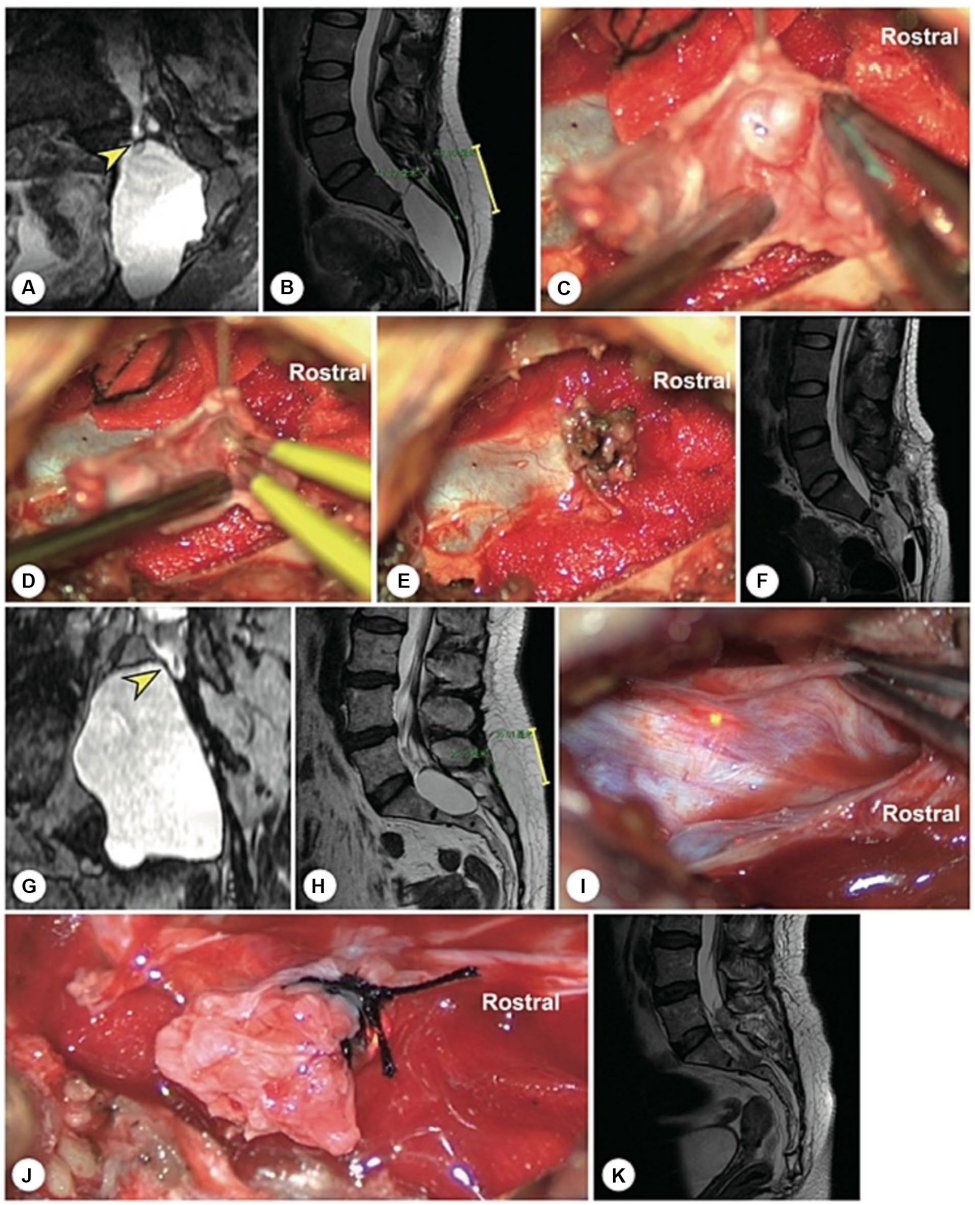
A representative case of an arachnoid hernia-type cyst and a representative case of a spinal dura mater leakage cyst. (A) Preoperatively, reconstructed MR image revealing an arachnoidal structure within the cyst (the yellow arrowhead indicates the cystic leakage orificium). (B) A 4 cm precise and minimally invasive incision was made (yellow line segment). (C–E) Intraoperatively, an arachnoidal diverticulum was found as the origin of the cerebrospinal fluid, and this cystic leakage orificium was ligated. (F) Follow-up MRI showed that the cyst had disappeared and that there was no recurrence. (G) Preoperatively, reconstruction MRI showed that the narrow end of the dural sac was squeezed to the left by a giant cyst without nerve roots (the yellow arrowhead indicates the cystic leakage orificium). (H) A 4 cm precise minimally invasive incision was made (yellow line segment). (I) Intraoperatively, we found that the lateral wall of the dural sac was weak, leading to leakage. (J) The cyst wall was dissected and turned over. (K) Follow-up MRI showed that the cyst had disappeared and that there was no recurrence.
